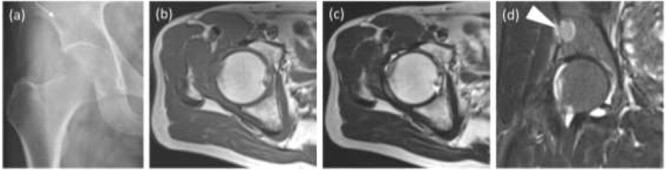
Images after the arthroscopic surgery. (a) The radiograph showed no calcification, which was observed before the arthroscopic surgery, and a stronger radiolucent shadow of the pelvic bone (an arrow). (b) T1WI axial, (c) T2WI axial, (d) coronal short T1 inversion recovery (STIR) sequences did not show the intra-articular lesion and the pelvic lesion got larger (a headless arrow).
